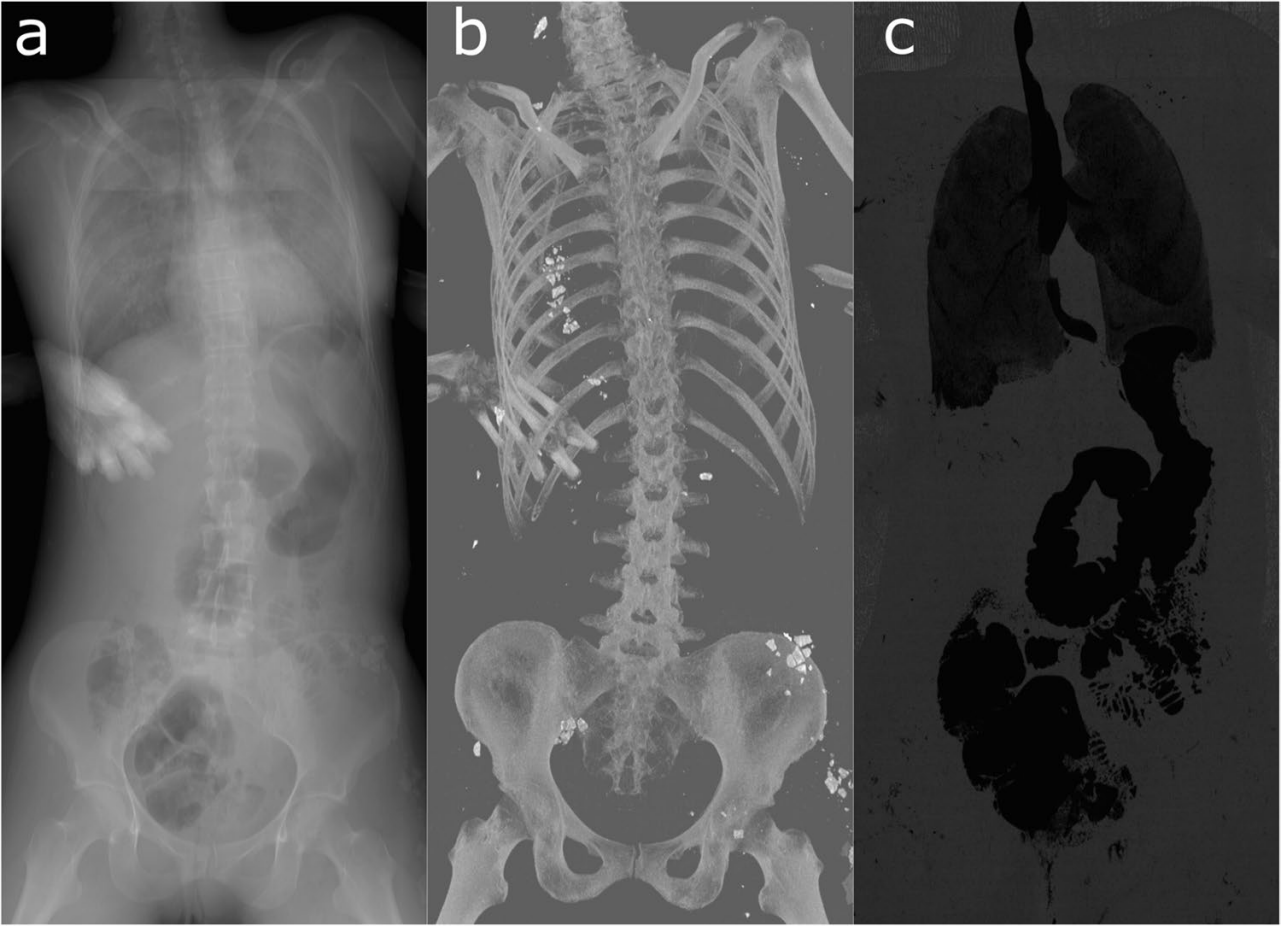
Different volume visualizations of the same PMCT dataset. a Averaging visually mimics the appearance of a plain radiograph. b MIP highlighting structures with high x-ray density such as bone. The high-density material visible in this image is debris that is located underneath the body. c MinIP visualizing the gas distribution inside the body and excluding the air surrounding the body
